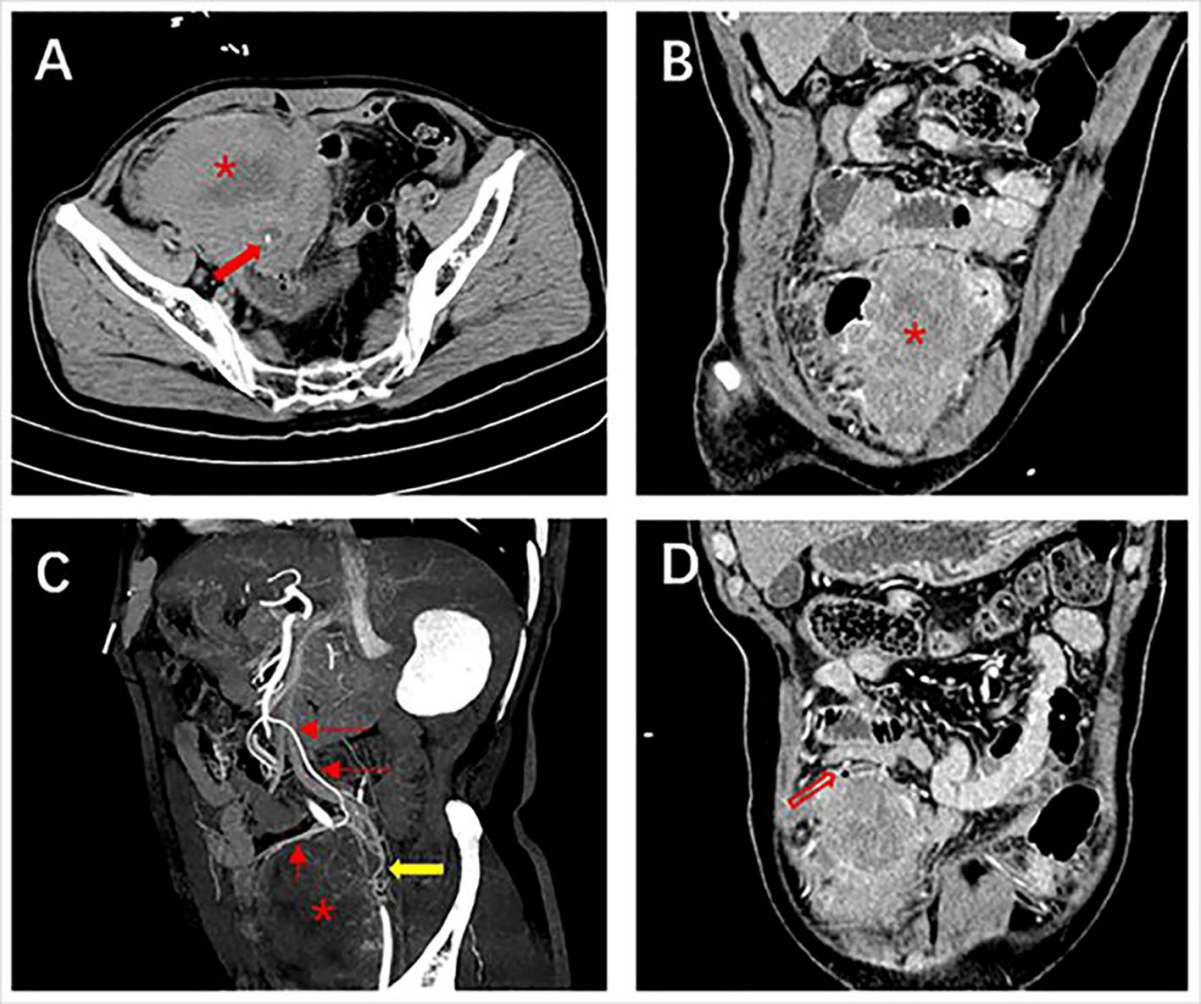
Abdominal contrast-enhanced computed tomography of case one. (A) Plain scan shows a large heterogeneous mass (*) with calcification (red solid arrow) at the margins at the end of the ileum measuring 8.7×7.0 cm; (B) Adjacent bowels are pushed by the exophytic, heterogeneous enhanced mass; (C) The artery supplying blood to the mass is from a branch of the superior mesenteric artery and a tortuous draining vein is seen (yellow arrow); (D) Free gas in the abdominal cavity (red hollow arrow).
